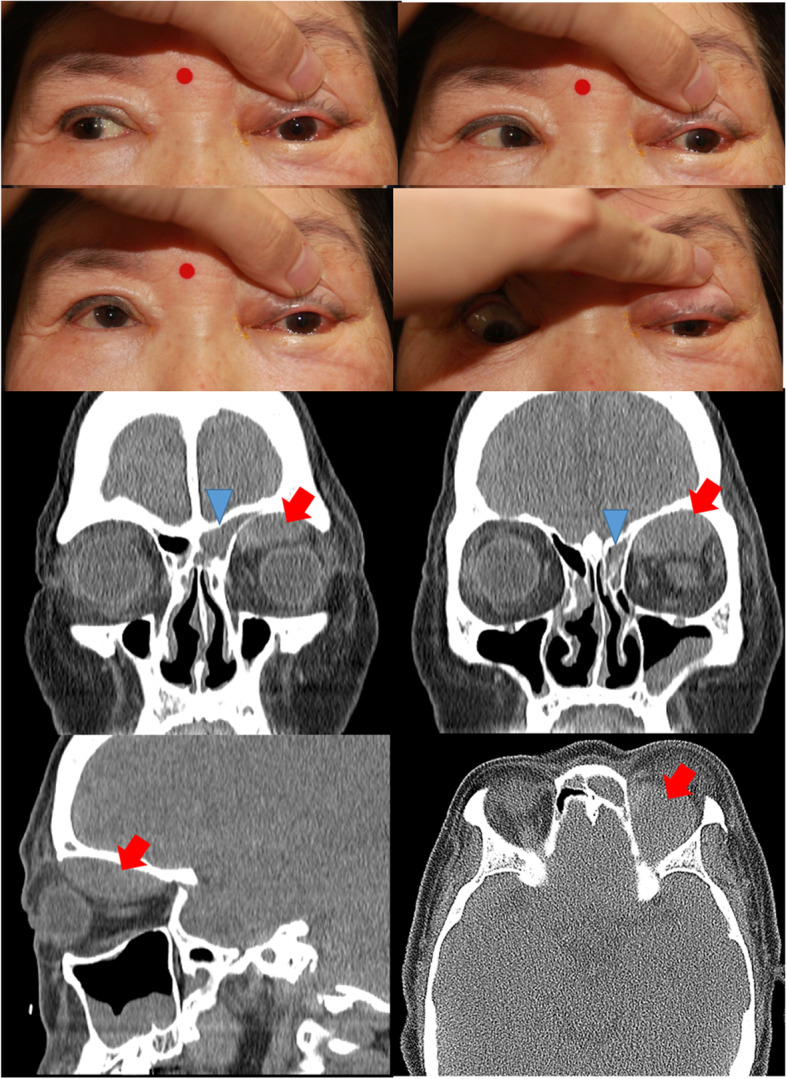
Limited extraocular muscle movement in terms of elevation, depression and adduction of the left eye. A well-demarcated biconvex non-enhancing soft tissue density lesion can be seen in the left superior subperiosteal area on non-enhanced CT
